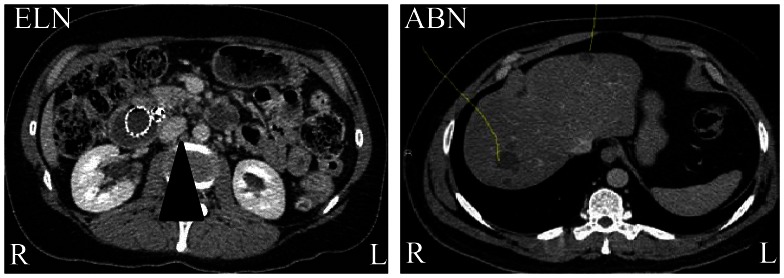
Cross-sectional abdominal CT-images used in the experiment. The CT-images depict an enlarged lymph node from an ELN-video (arrowhead in a) and liver cysts from an ABN-video (yellow lines in b). Note that the left and right side of the body are switched in the images.
